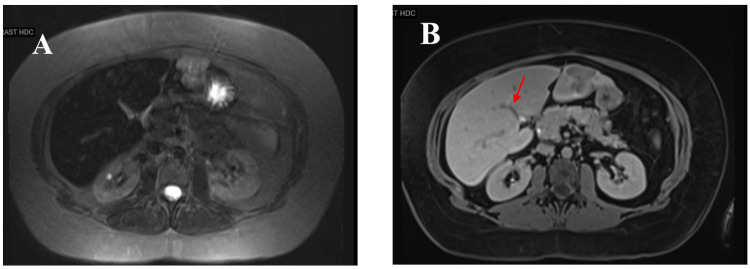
MRI of the liver left lobe of a 31-year-old woman showing a heterogenous, irregular, hyperintense mass on T2 in segment 3 (A) Five minutes after contrast injection, the lesions are not visible. (B) Twenty minutes after contrast injection, there are multiple, hyperintense lesions on T2 indicated by a red arrow, consistent with intrahepatic cholangiocarcinoma.
